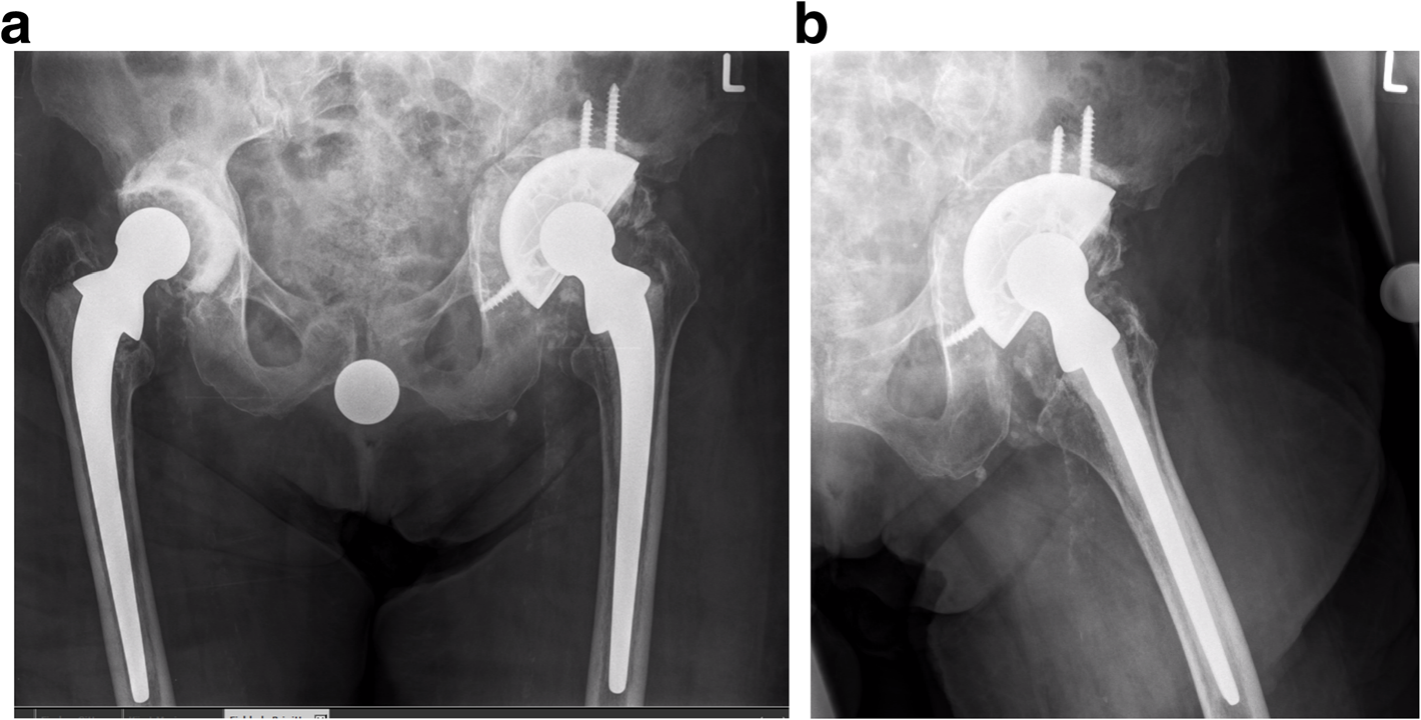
a Allograft filling with the distraction technique. The defect was stabilized with a cementless revision shell (REDAPT size, 78 mm, locking screws cranial and distal). Reconstruction of the center of rotation with the reverse augmentation technique. b Lateral view of the postoperative X-ray
